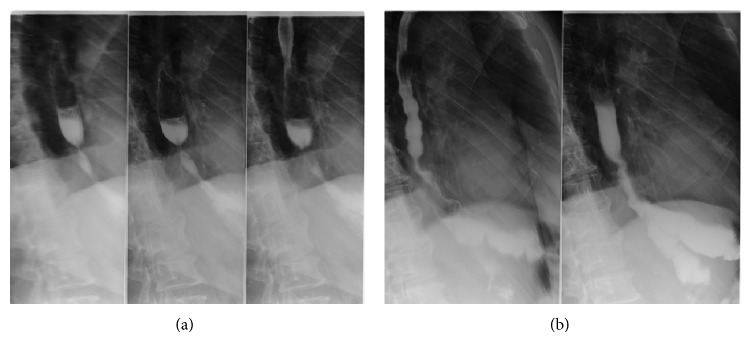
Abdominal X-ray: before (a) and after (b) balloon dilatation.
